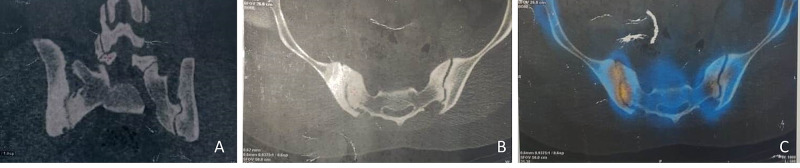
Computed tomographic scan (A, B) and the nuclear scan showed sacroiliac arthritis and increased uptake in the right sacroiliac joint
